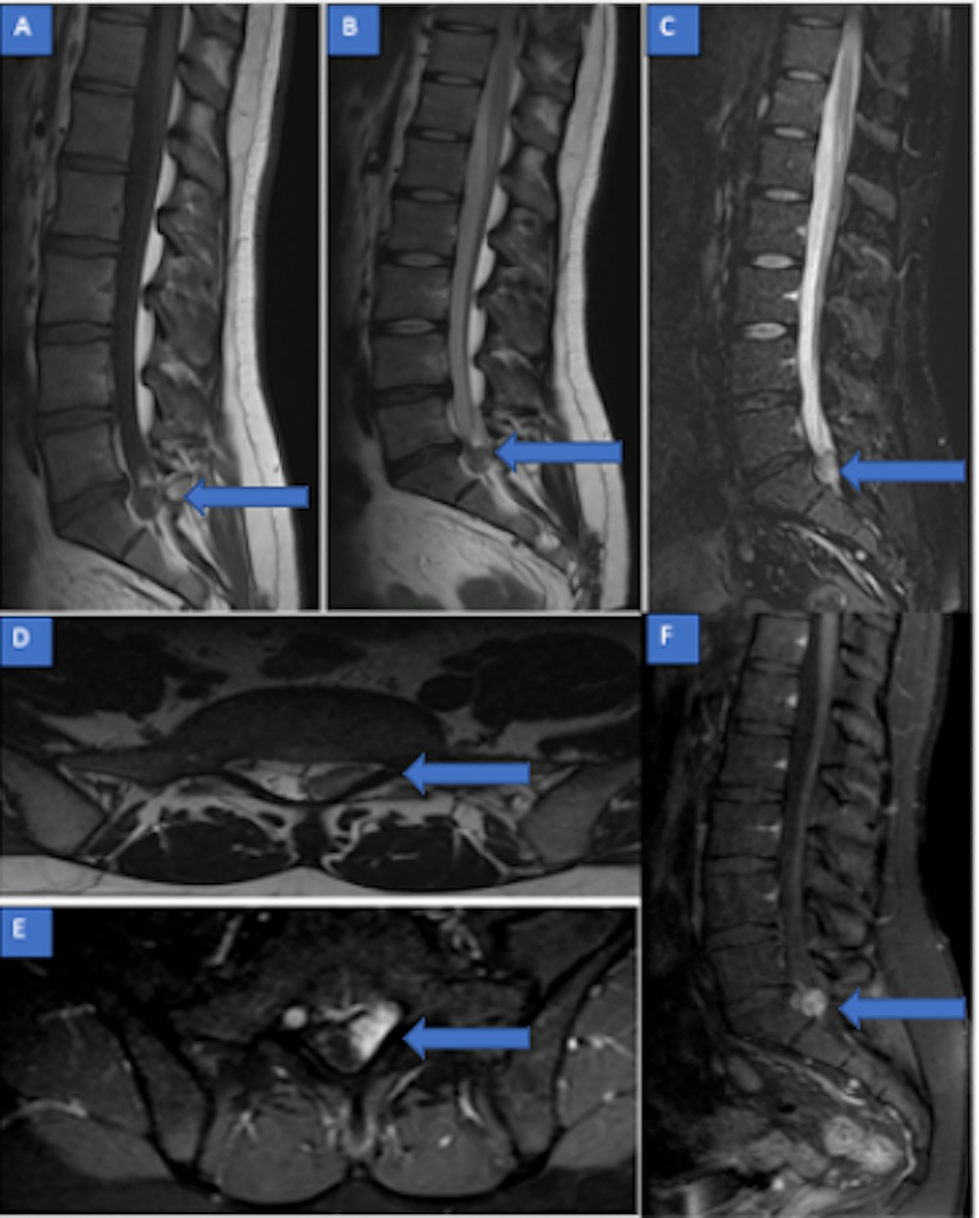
Lumbar spine MRI (November 2019) (A) Sagittal T1, (B) T2, (C) T2 STIR, (D): axial T2: intracanalar expansive mass of 14 mm, located in the left posterolateral epidural space at the S1 level, hypointense on T1-WI, heterogeneous on T2-WI and hyperintense on T2 STIR-WI. (E, F) Axial and sagittal T1 with gadolinium: homogeneous and intense enhancement of the whole lesion. MRI - Magnetic Resonance Imaging
